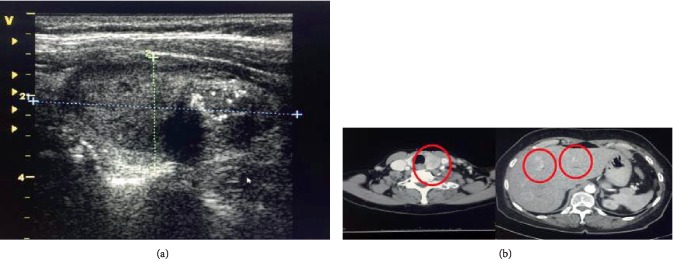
(a) Thyroid ultrasound demonstrating hypoechoic lesion. (b) Preoperative CT of the neck (left) and the abdomen (right) revealing cervical lymphadenopathy and liver metastasis.
